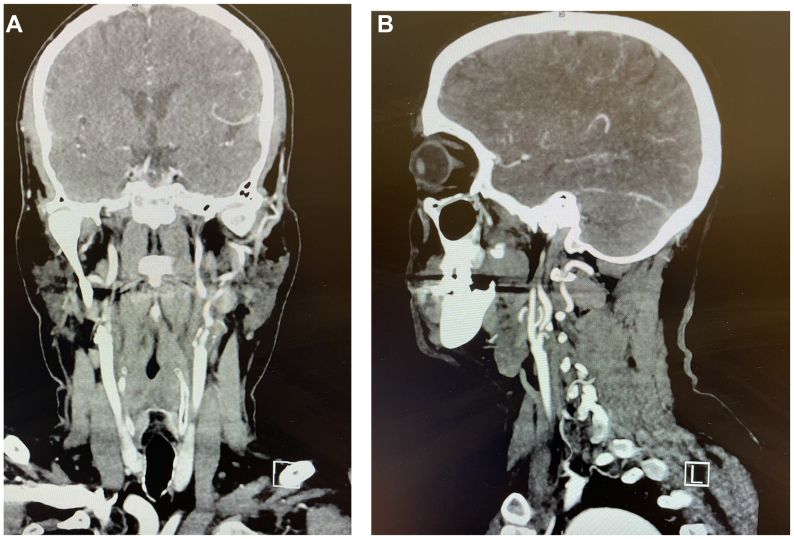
(A) This computed tomography angiogram of the neck highlights the high-grade stenosis in the proximal left internal carotid artery (ICA). (B) Sagittal view of the significant narrowing of the artery suggesting a high risk of embolic events, which could lead to vision loss in the ophthalmic artery branches.
